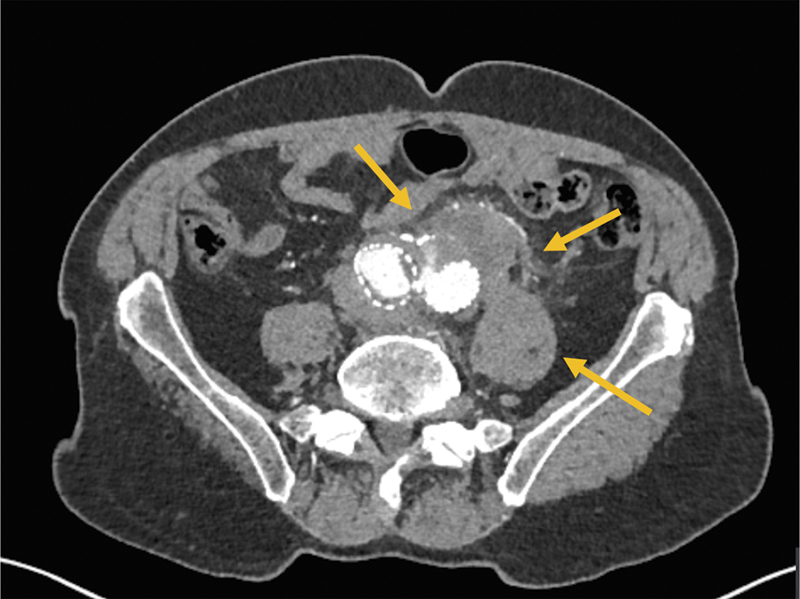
Axial view of the preoperative contrasted computed tomography of the aorta demonstrating a Type-II endoleak and a periaortic gas collection (highlighted by yellow arrows).
